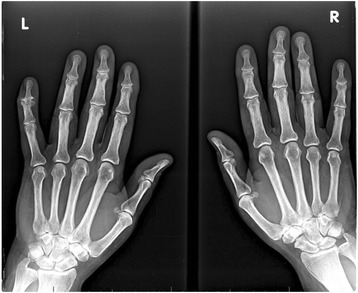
Plain films of both hands
